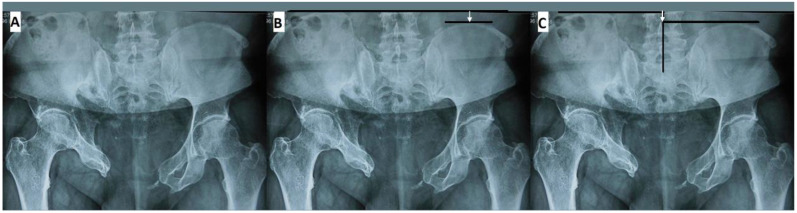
(A) Anteroposterior radiograph of a pelvic malunion with cranial vertical displacement of the right hemipelvis; (B) anteroposterior radiograph demonstrating measurement of vertical displacement of the right hemipelvis compared to the left (white arrow); (C) anteroposterior radiograph demonstrating measurement of vertical displacement of each hemipelvis along perpendicular vertical reference line (white arrow).
